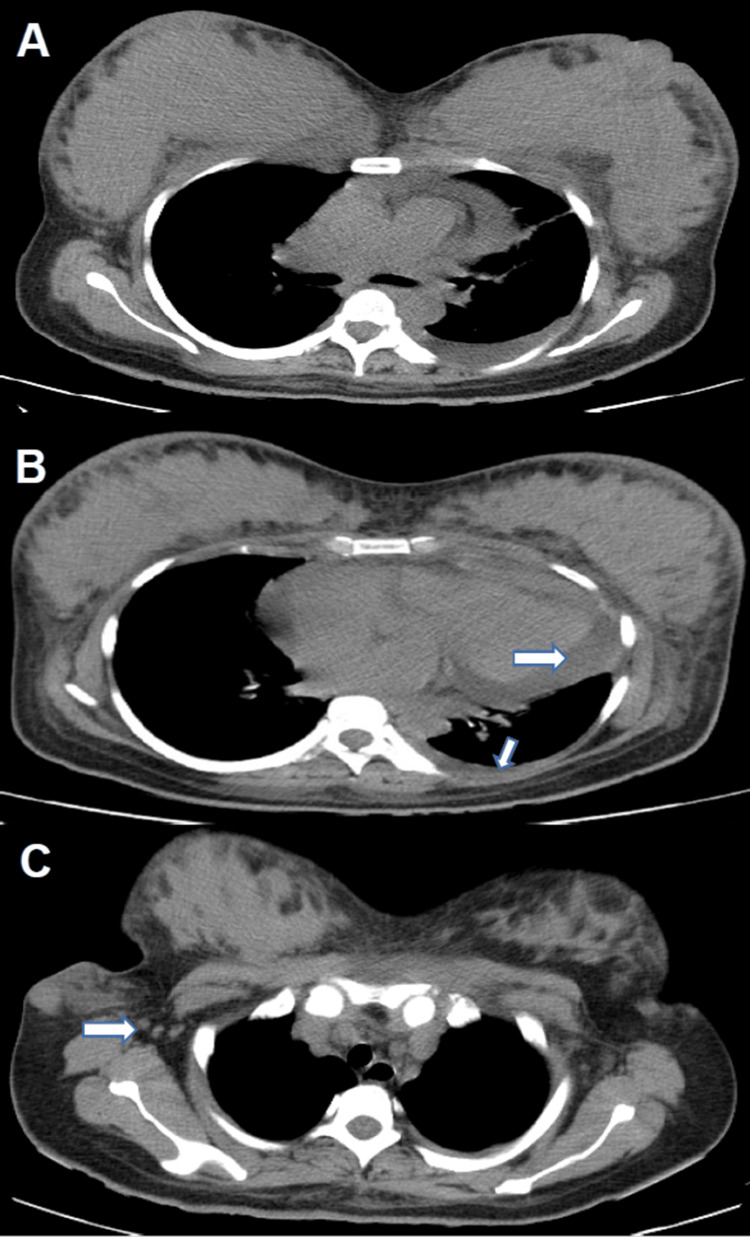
Chest CT A) diffuse bilateral mammary gland inflammation and swelling; B) a small amount of pericardial and pleural effusion (arrows); c. enlarged lymph nodes are seen in the right axillary area (arrows)
